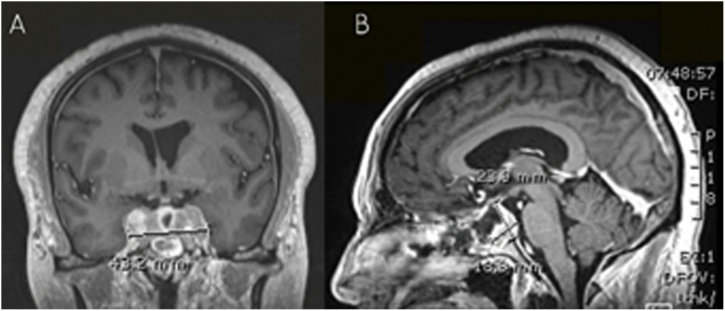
MRI brain T1 A) coronal view B) sagittal view showing sellar and suprasellar enhancing lesion replacing the pituitary gland with extension along the pituitary stalk and invasion of the cavernous sinuses measuring 4.3 × 2.3 × 1.8 cm.
